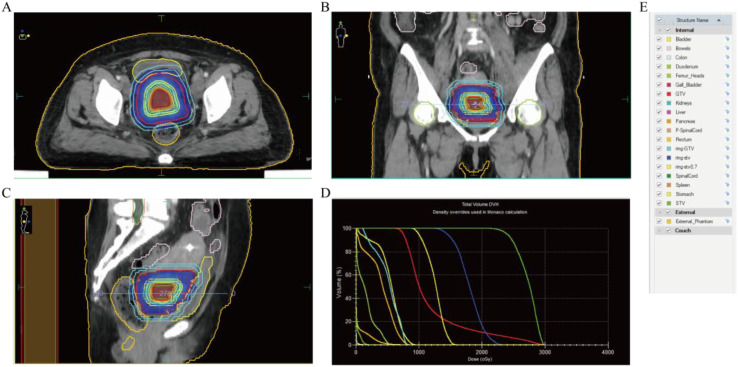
SBRT target volumes and dosimetry. GTV (Gross Target Volume) represents the overall anatomical range of the visible primary cervical tumor and metastatic sites on the CT images of this treatment plan, with a volume of 226.16 cm^3^. STV (Simultaneous Integrated Boost Target Volume): within the GTV, the core area most likely to be rich in active tumor cells determined by clinicians based on imaging characteristics, volume 13.16 cm^3^. (A) The radiotherapy target volume for the cervical lesion (axial view). (B) Radiotherapy target volume for the cervical metastases (coronal view). (C) Radiotherapy target volume for the cervical metastases (sagittal view). (D) Dose-volume histogram of radiotherapy plan. (E) List of organs at risk and target volume structures in the radiotherapy plan. Nevertheless, the bladder appeared flattened on the planning CT (3A) due to direct compression by the large cervical tumor, rather than inadequate filling. Bladder filling was monitored throughout radiotherapy using image guidance.
